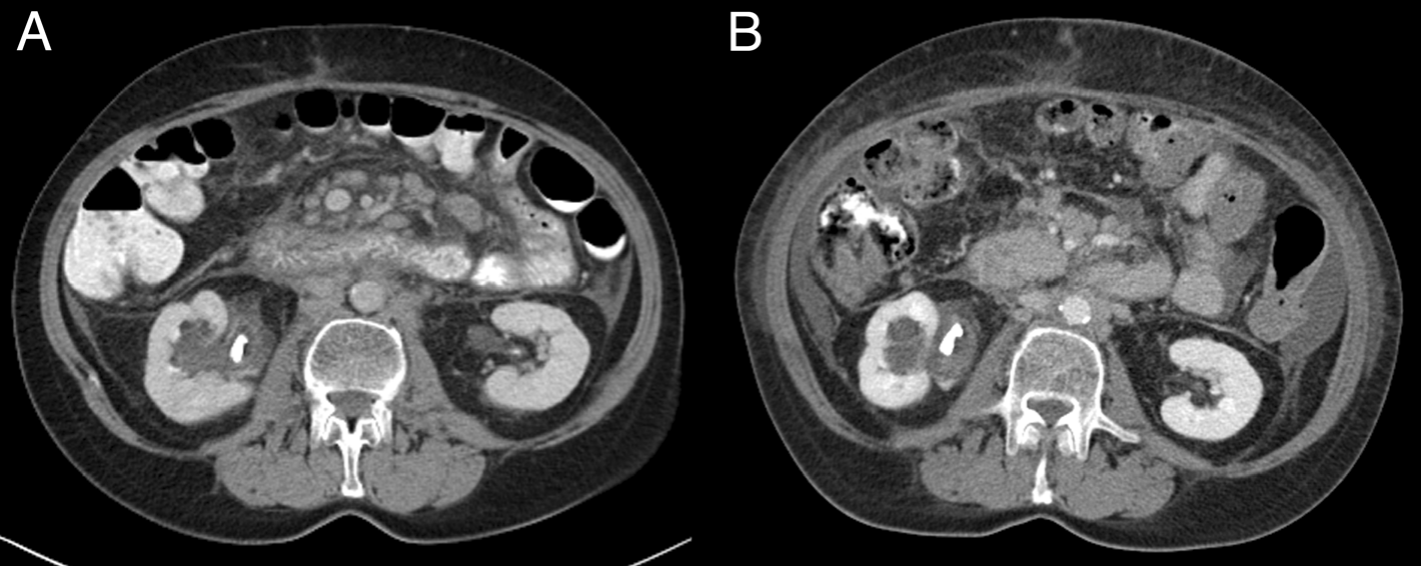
Image quality comparison in para-aortic lymph-nodes. Same patient in progressive disease. a Flash-CT: CTDIvol 7.72 mGy, DLP 516 mGy · cm. b Force-CT: CTDIvol 3.88 mGy, DLP 278.5 mGy · cm
